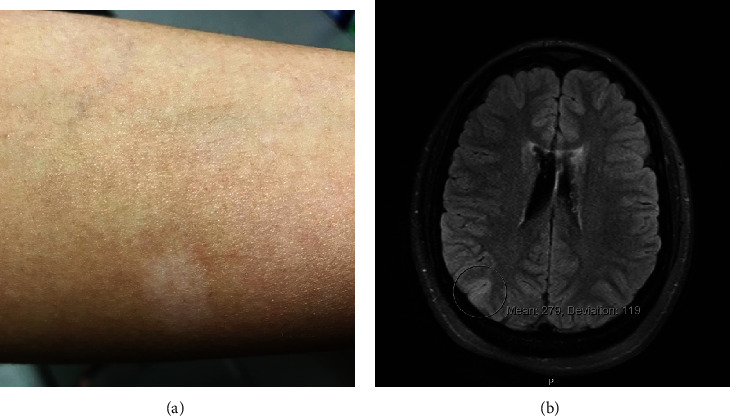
(a) External color photograph demonstrating a cutaneous ash leaf spot on the patient's forearm. (b) MRI of the brain showing FLAIR hyperintensities involving the right posterior parietal lobe demonstrating a cortical tuber (circled).
